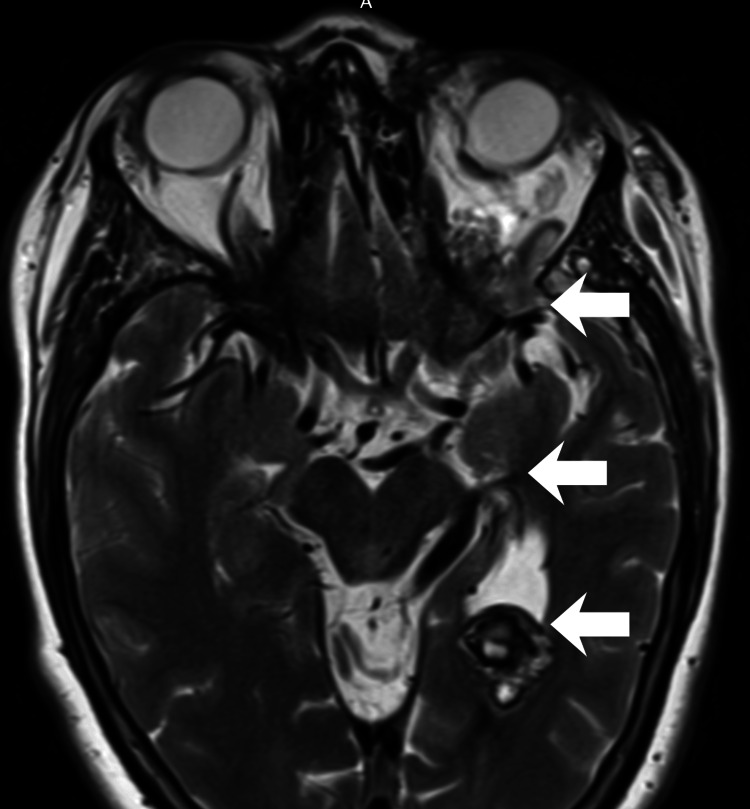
Deep venous connection and intraventricular cavernoma T2-weighted image demonstrating dilated superficial medial cerebral veins and basal vein of Rosenthal, connecting to a heterogeneous lesion with hemoglobin degradation products in the posterior aspect of the left lateral ventricle occipital horn, compatible with a cavernoma.
